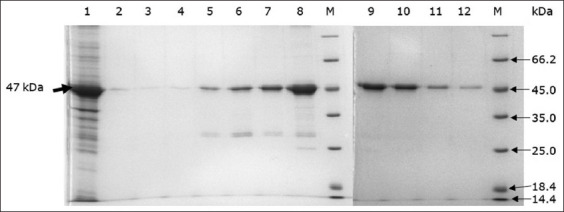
Sodium dodecyl sulfate-polyacrylamide gel electrophoresis of rP32Trx protein purified using metal affinity chromatography. Lane 1: Supernatant of the *Escherichia* coli BL21(DE3)/P32Trx after ultrasonication, Lane 2: Wash buffer, Lanes 3 and 4: 50 mM imidazole eluent, Lanes 5 and 6: 100 mM imidazole eluent, Lanes 7 and 8: 150 mM imidazole eluent, Lanes 9–12: 500 mM imidazole eluent, Lane M: Molecular weight markers (#26610, Thermo Fisher Scientific).
